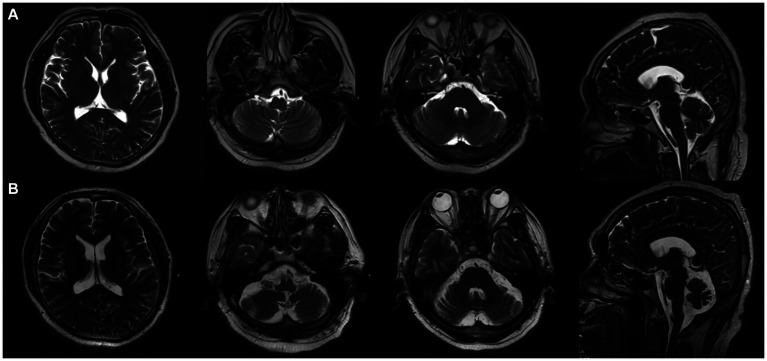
On 25 March 2020 (A), cranial MRI showed no abnormality, and on 20 April 2023 (B), cranial MRI showed cerebellar atrophy.
